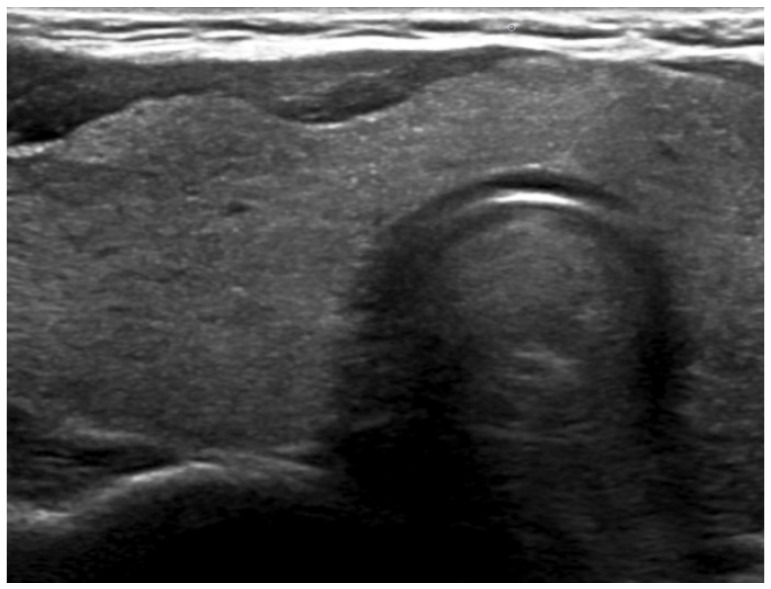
Ultrasound in the region of the right lobe of the thyroid gland.
